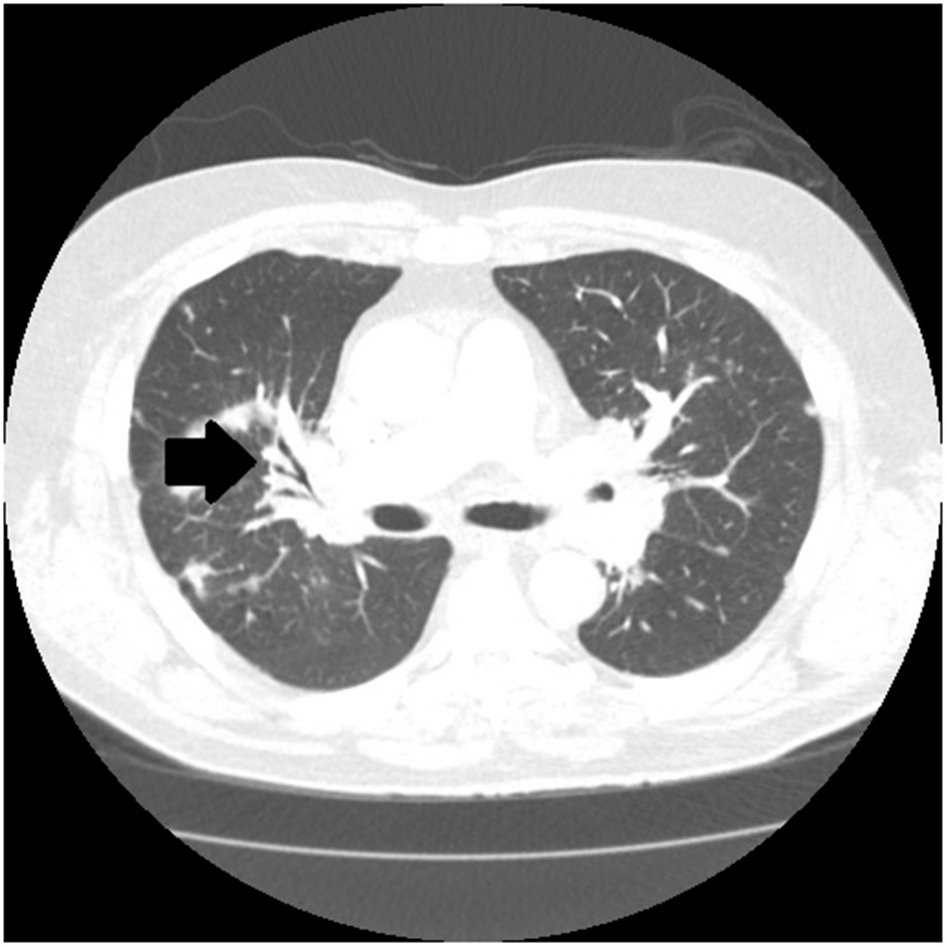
Chest CT scan showing sarcoidosis nodules coalescing around the bronchovascular bundle (arrow).
